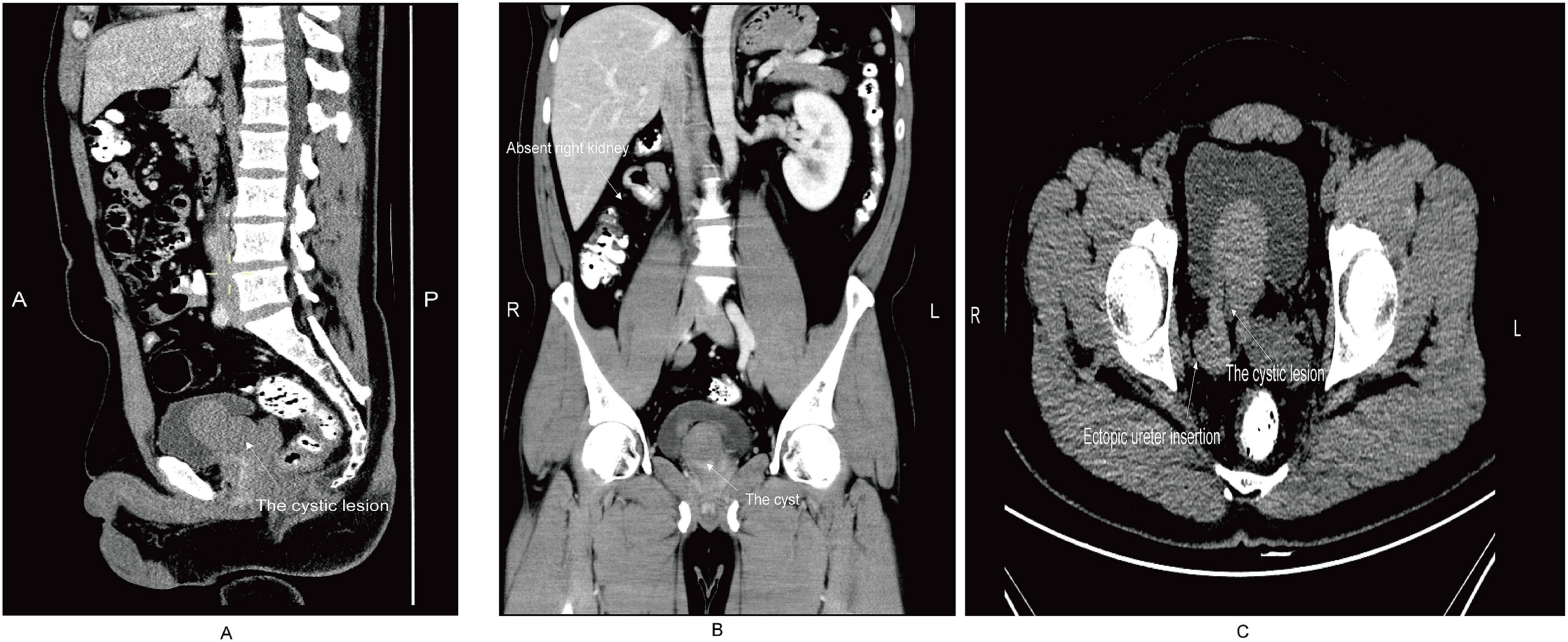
Contrast-enhanced computed tomography (CT) findings of the patient. (A) Sagittal CT image demonstrating a cystic lesion arising from the right seminal vesicle, protruding into the bladder cavity (arrow). (B) Coronal CT image showing right renal agenesis and a well-defined cystic mass located posterior to the bladder, consistent with a seminal vesicle cyst (arrow). (C) Axial non–contrast-enhanced axial CT image revealing an ectopic insertion of the right ureter into the right seminal vesicle, with the cyst partially protruding into the bladder lumen (arrows).
